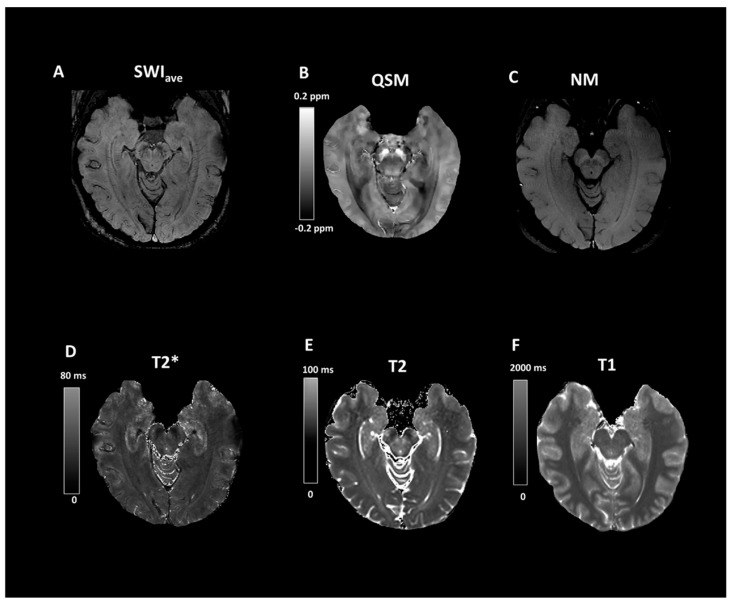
Representative images of (A) multi-echo susceptibility-weighted image (SWI_ave_), (B) quantitative-susceptibility mappings (QSM), (C) neuromelanin-sensitive MRI (NM-MRI), (D) T2* map, (E) T2 map and (F) T1 map for a 20-year-old female healthy subject.
